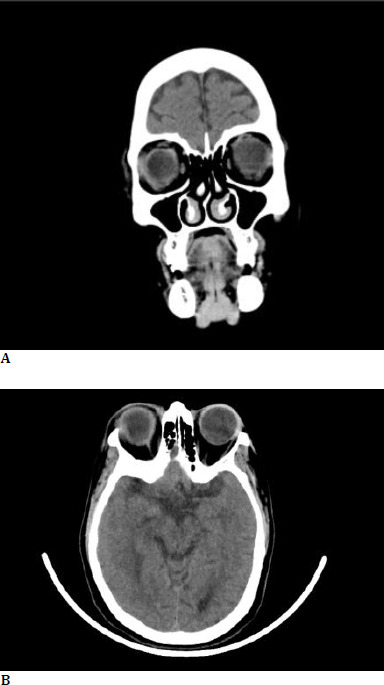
Computed tomography image - coronal section showing an inflamed lacrimal gland and bulky extraocular muscles; B. Computed tomography image - axial section showing an inflamed lacrimal gland
